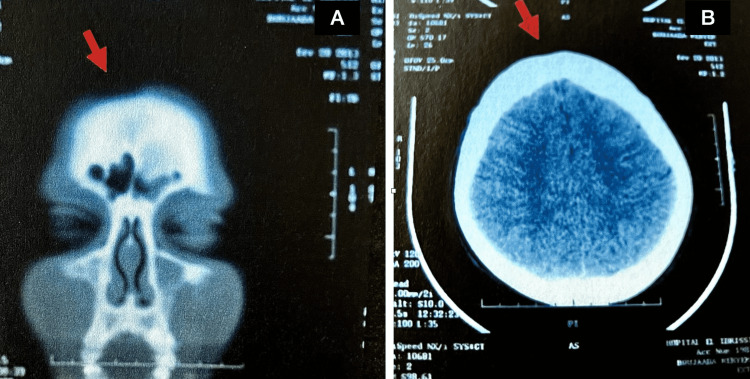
CT scan of patient 2 with no bone invasion (A) Coronal section and (B) axial section. Arrow: discrete frontal depression.
